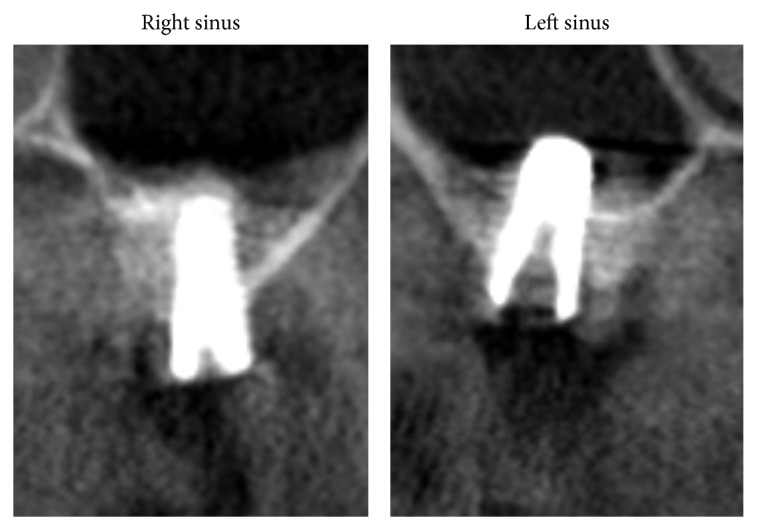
CBCT exam at 5 years. Note the absence of Schneiderian membrane thickening on the coronal view of implant placed with grafting material (right sinus, second molar site) and without grafting material (left sinus, second molar site). The CBCT confirms that the implant in the left sinus protruded into the sinus whereas the implant in the right sinus was completely embedded in peri-implant bone.
